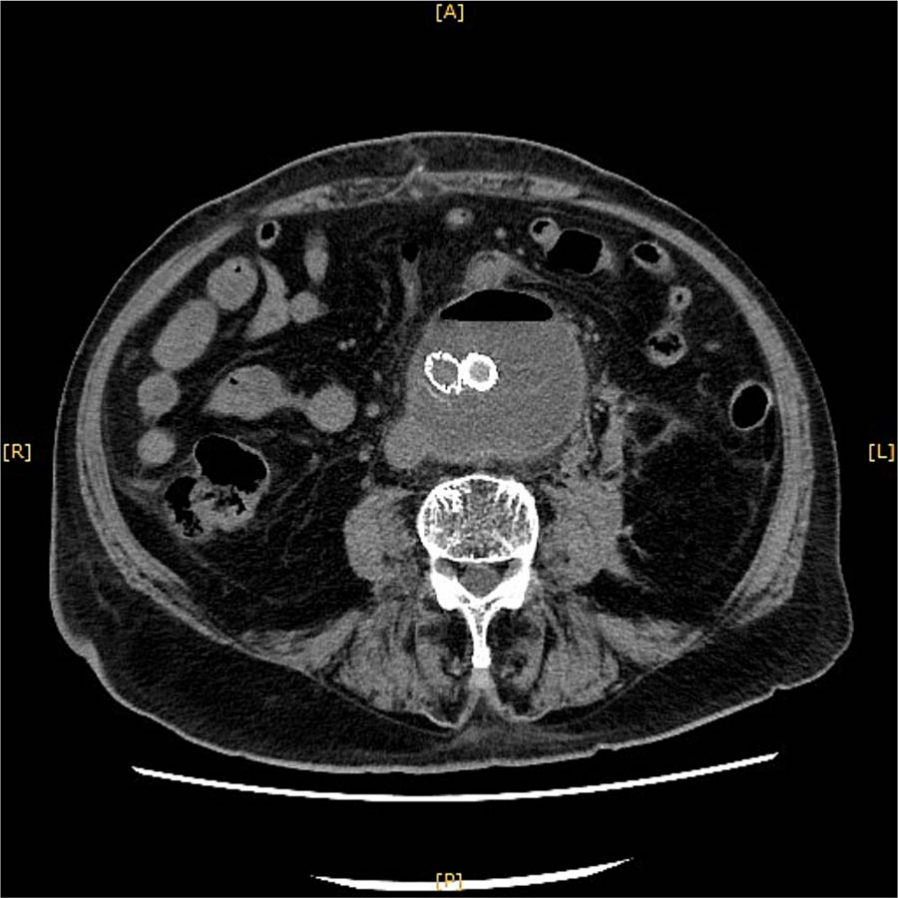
A plain CT on POD 14 showed a reduction in the aneurysm size with no apparent leaks.
